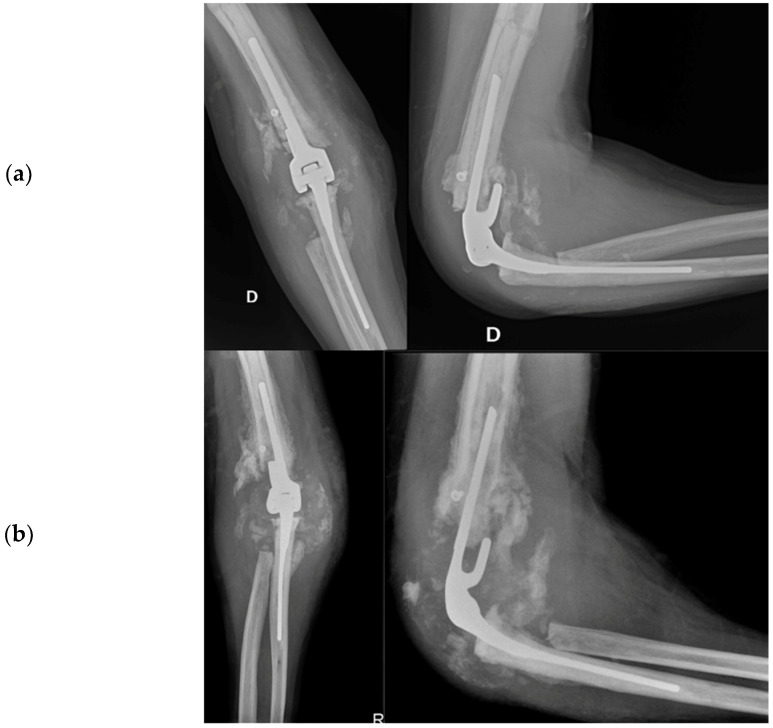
(a) Radiographic X-ray anteroposterior and lateral views: beginning of prosthesis loosening with increased bone radiolucency around the prosthesis; (b) 30 days after loosening of the prosthesis and increasing of periarticular calcifications.
